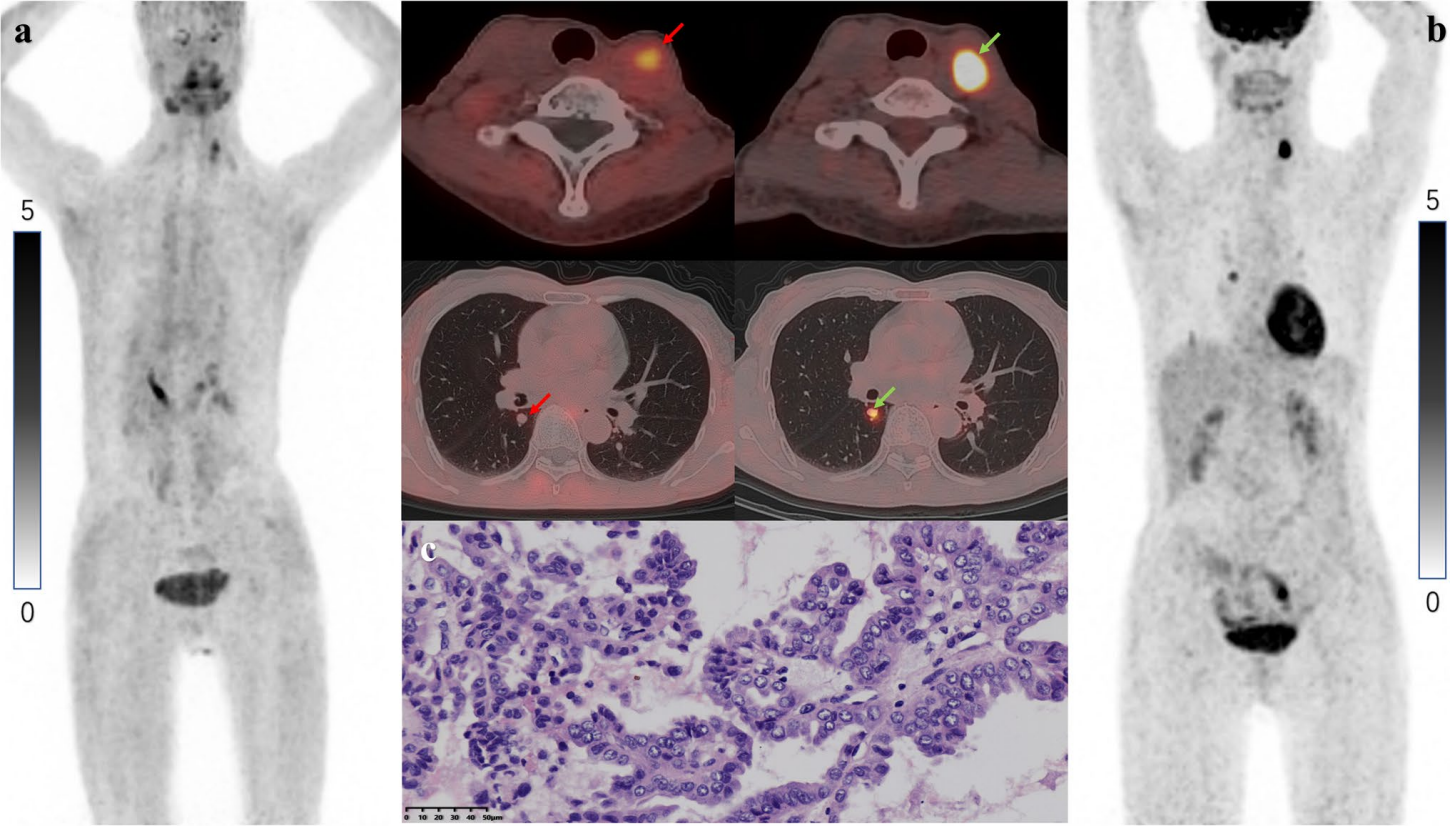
Metastatic lesions in a 67-year-old woman. a [^18^F]FAPI-42 PET/CT revealed local lymphatic and pulmonary lesions with low-to-moderate [^18^F]FAPI-42 uptake (lesions indicated by red arrows on axial fusion images). b The uptake of 2-[^18^F]FDG was higher than that of [^18^F]FAPI-42 (lesions are indicated by green arrows on axial fusion images). c Pathologically confirmed diagnosis of PTC via US-FNA
